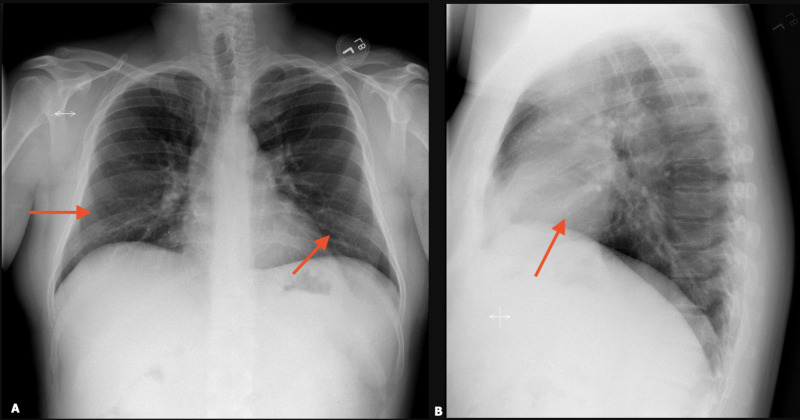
Follow-up chest x-ray two weeks following discharge showed almost complete resolution of bilateral airspace opacities with near normal lung parenchyma (red arrows) A) Postero-anterior view; B) lateral view
